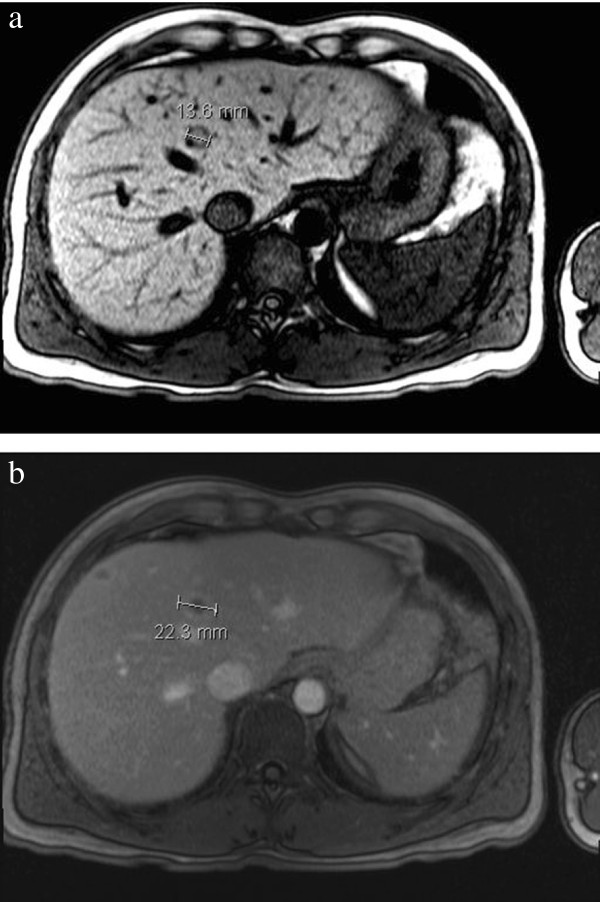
Characterization by MRI. (a) Lesion with hypointense rim and isointense centre; (b) Lesion with centrally low signal intensity and rim enhancement.
